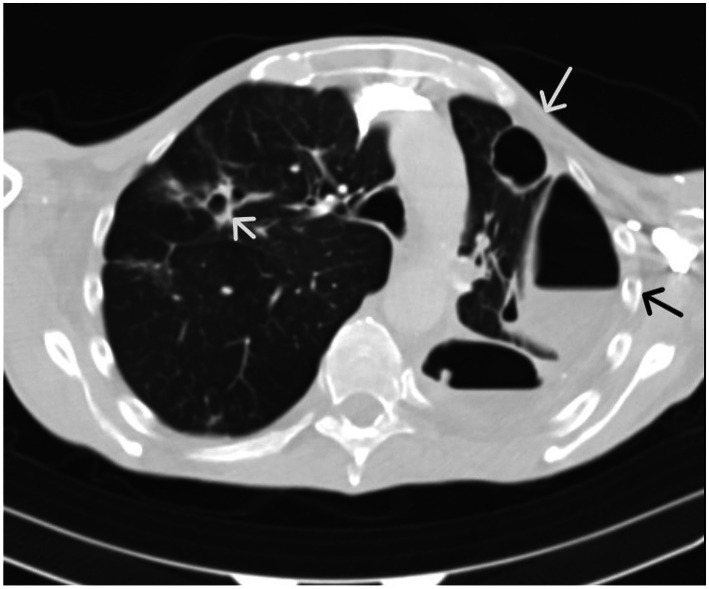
Lung computed tomography with bilateral excavations and left-sided hydropneumothorax.
